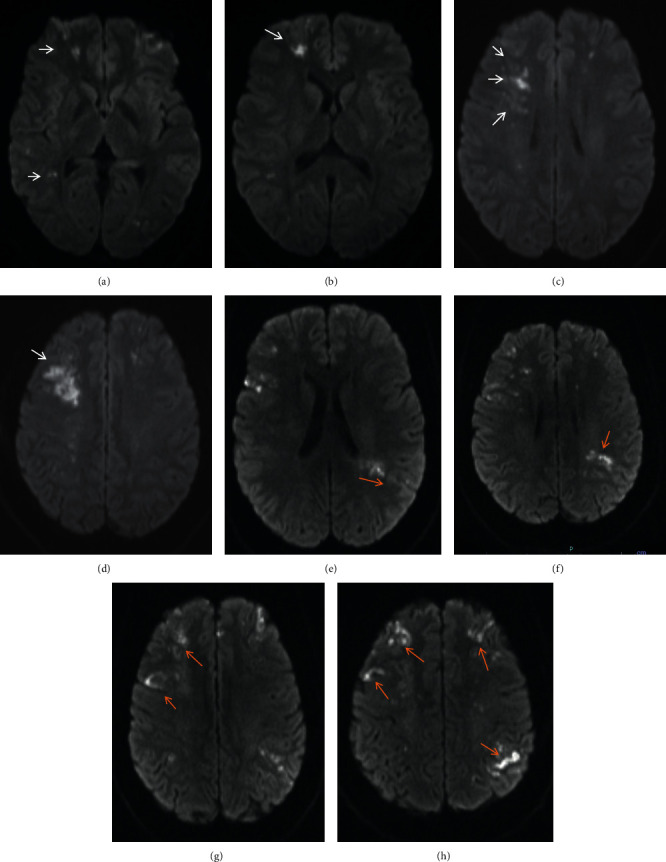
Axial MRI brain imaging: (a)—(d) images from the first episode of stroke, with regions of restricted diffusion indicative of acute ischemia (arrows), primarily noted in the right cerebral hemisphere; (e)—(h) images from the second episode, with progressive bilateral ischemic changes (arrows).
